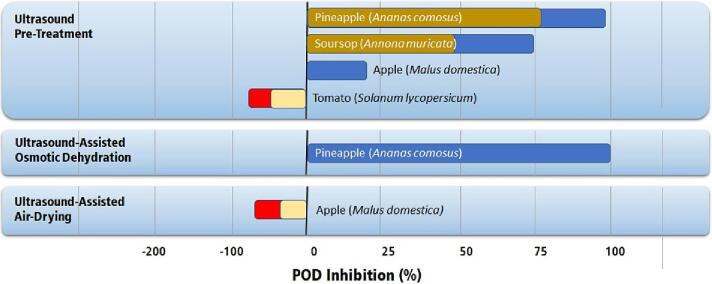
Highest inhibition of POD in fruits by applying ultrasound technologies [25], [99], [156], [157], [160], [161], [162], [163], [164]. Single-colored bars indicate unique values in the literature. Double-colored bars indicate the lowest (brown and yellow) and highest (blue and red) values reported in the literature.
